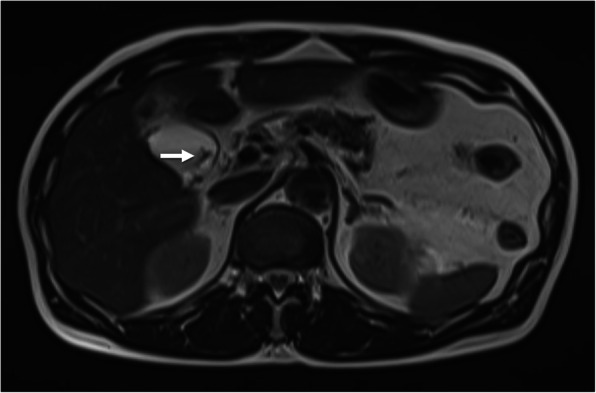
Representative image from the T2 axial MRI. A pedunculated polypoid lesion was observed in the neck of the gallbladder
